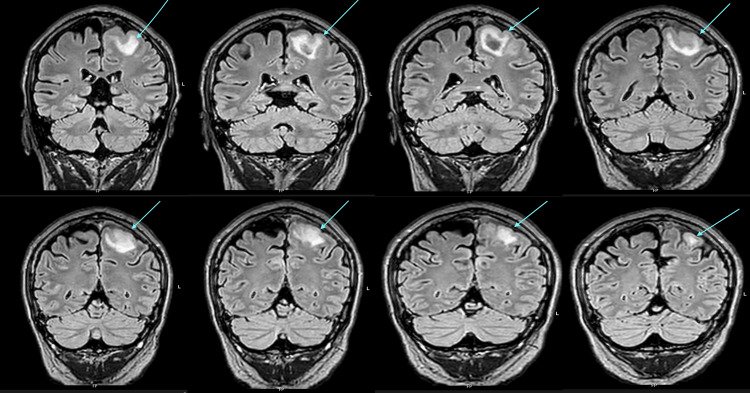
MRI brain (FLAIR (Fluid-Attenuated Inversion Recovery) sequences) confirms a left frontal parenchymal haemorrhage (blue arrow), with minor diffusion restriction and perifocal oedema.
